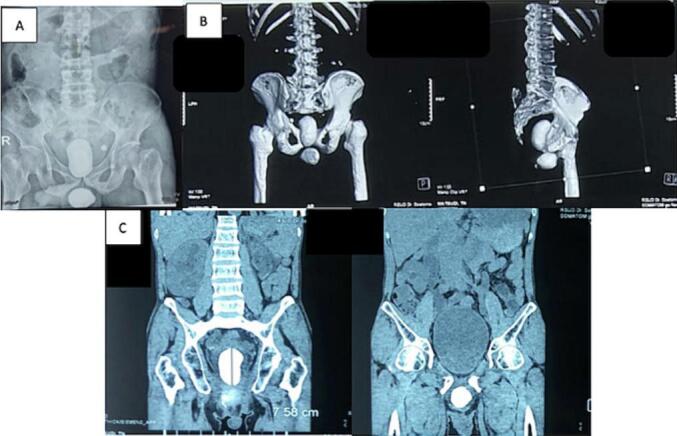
(a) KUB Photo; (b) 3D reconstruction of CT-Stonography; (C) Sagittal view of CT-Stonography.
